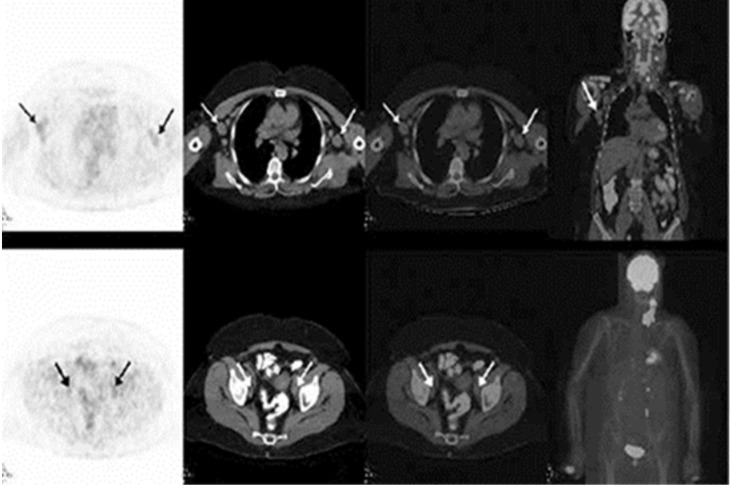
FDG-PET/CT images demonstrating minimally FDG-avid axillary and pelvic lymph nodes suggestive of indolent lymphoma A 64-year-old female with a diagnosis of CLL/SLL. Axial PET and fused axial and coronal PET/CT images demonstrated minimally FDG-avid right cervical, right supraclavicular, and bilateral axillary nodes (upper row), and minimally FDG-avid bilateral internal iliac nodes (lower row), which was compatible with the low FDG uptake of indolent lymphoma. (Reference: Yilmaz, et al. [21]).
